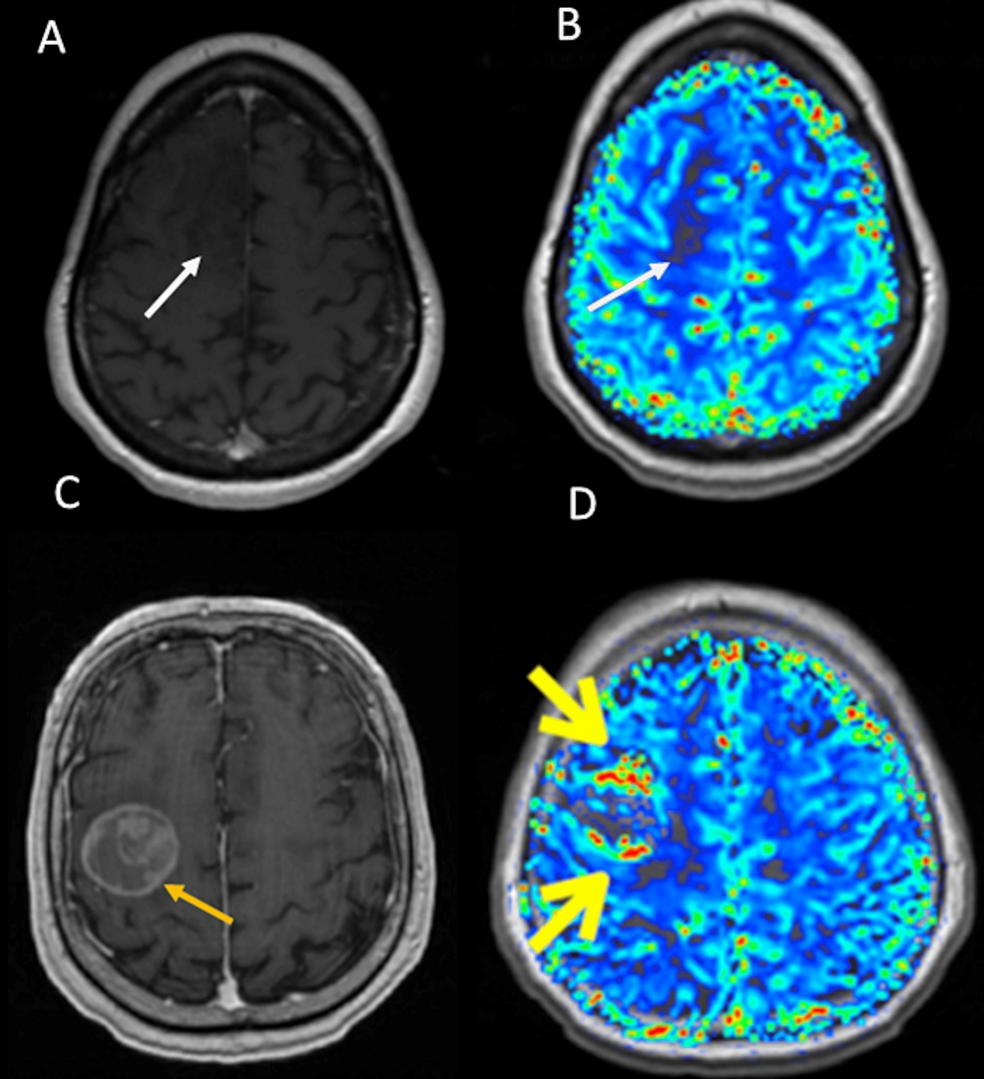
Role of DSC in differentiating between different brain tumors. (A, B) The images represent a right frontal lobe oligodendroglioma (white arrow). (C, D) The images represent right frontal lobe non-small cell lung cancer metastasis (yellow arrow). (A) Post-contrast T1-weighted MRI shows a non-enhancing right frontal lobe lesion. (B) Color-coded rCBV map from DSC data depicting no hyperperfusion. (C) Post-contrast T1-weighted MRI shows a lesion with heterogeneous enhancement. (D) Color-coded rCBV map from DSC data shows hyperperfusion.
